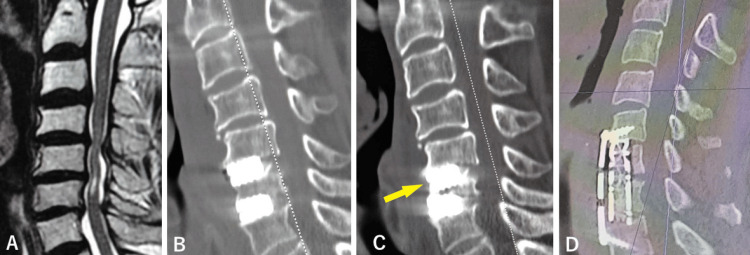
Case 1: Sagittal MRI and CT scan before and after two operations. T2-weighted MRI sagittal image before the first operation (A), sagittal reconstructed CT scans after the first operation showing that sufficient decompression was achieved (B), 37 days after the operation showing  that the cage at C5/6 had subsided 8 mm to the C6 vertebral body (C, arrow), after the second operation showing the proper position of the mesh and plate and good cervical spine alignment (D). MRI: magnetic resonance imaging
